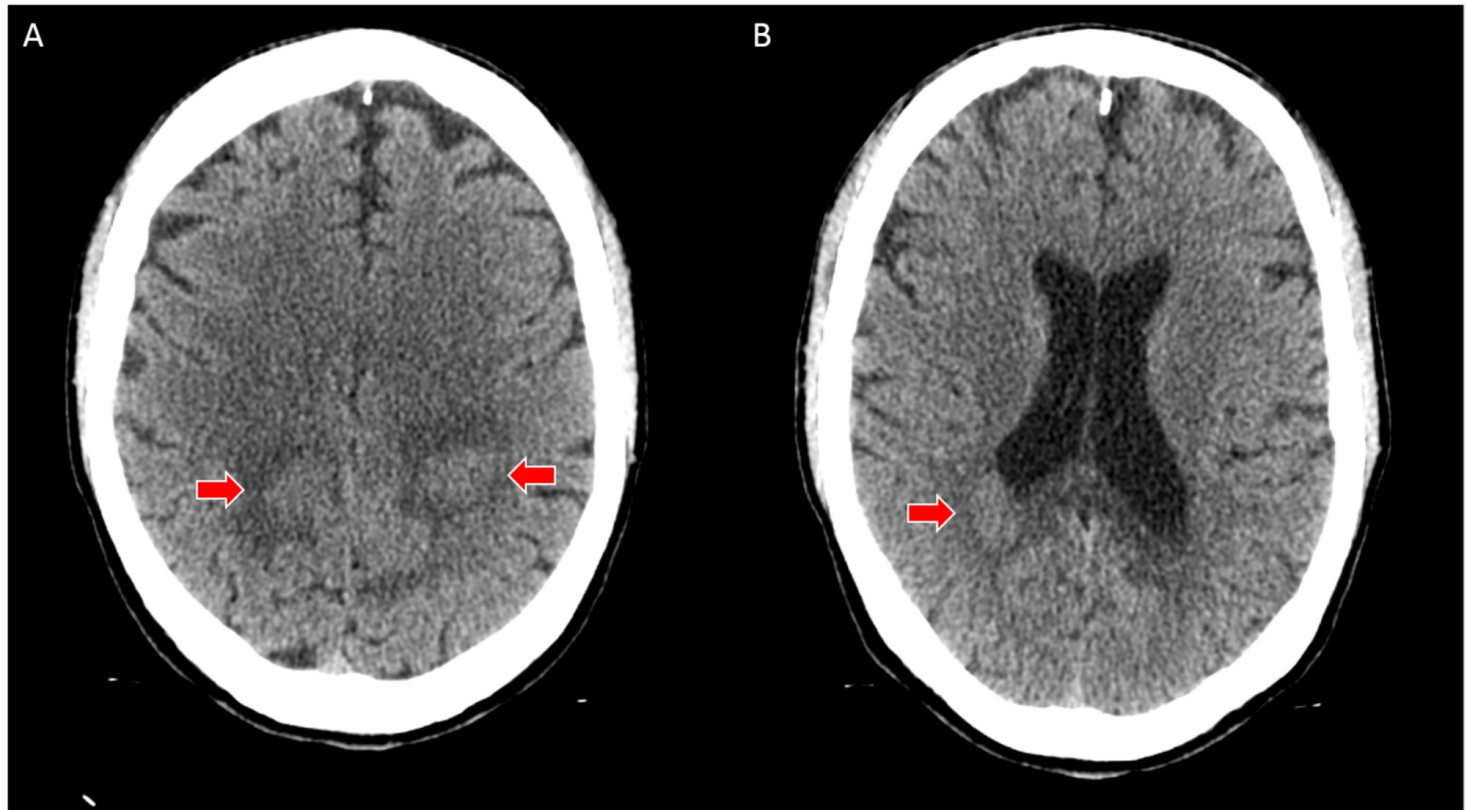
A - axial computed tomography (CT) image demonstrates a slightly hyperdense mass with adjacent edema involving the parietal white matter (red arrows). B - axial CT image demonstrates a slightly hyperdense mass with adjacent edema involving the splenium of the corpus callosum (red arrow)
